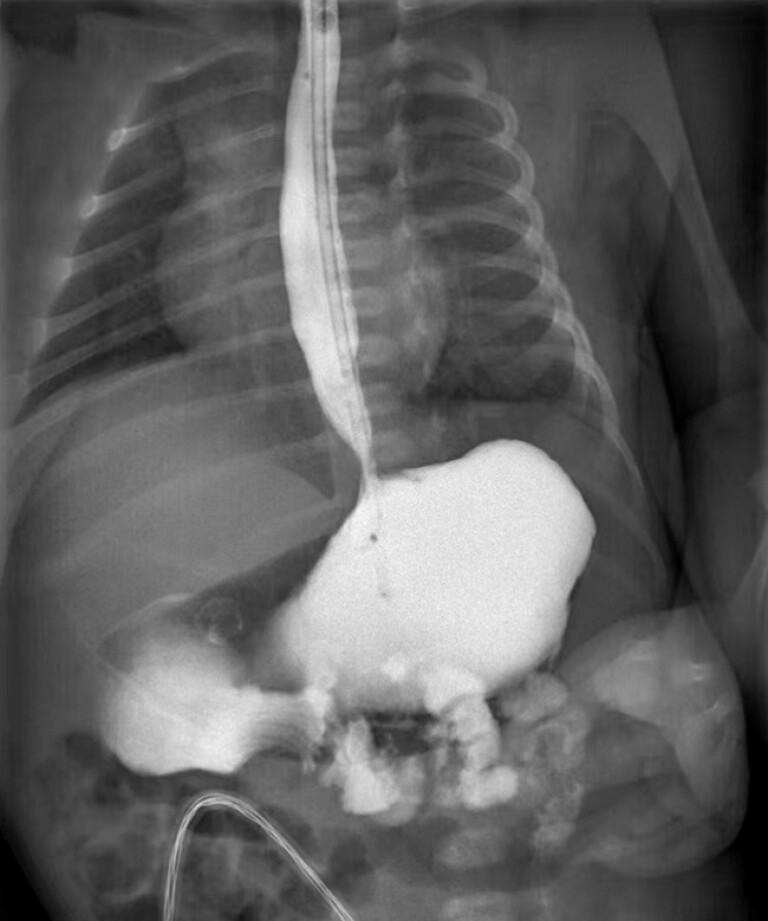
Upper gastrointestinal radiography showed the resolution of the obstruction.
